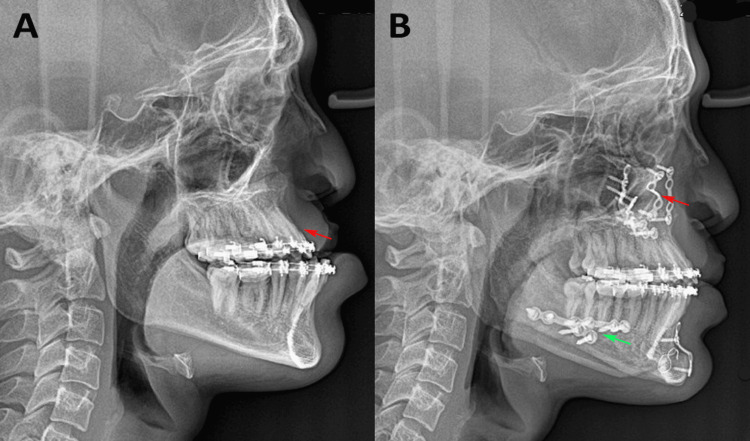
Bone-on-bone telescopic fixation technique. (A) Preoperative lateral cephalometric radiograph of a cleft lip and palate patient with maxillary hypoplasia and mandibular prognathism prior to surgery (red arrow indicating the maxillary hypoplasia); (B) postoperative lateral cephalometric radiograph demonstrating the bone-on-bone telescopic fixation technique following maxillary advancement using a step plate as visible at the maxillary osteotomy site (red arrow), along with mandibular setback surgery (green arrow). Original images of the patient, used with the patient's permission.
